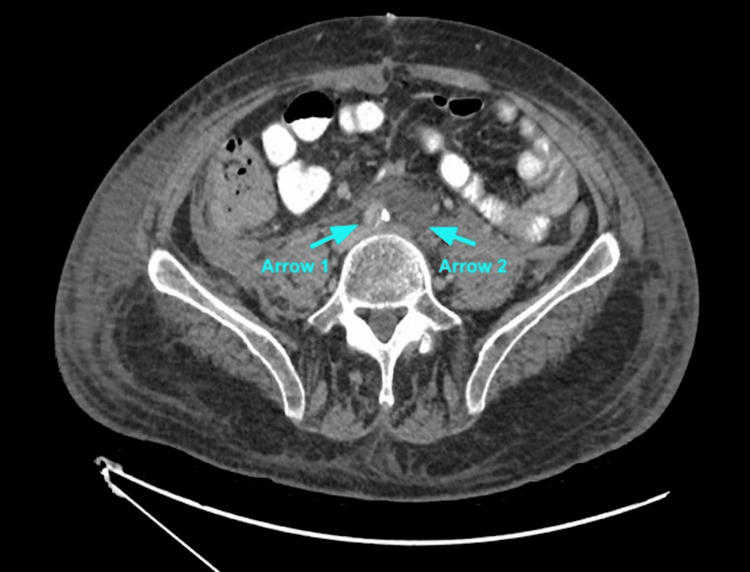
Severe Stenosis of the Right Common Iliac Artery and Occlusion of the Left Common Iliac Artery on CT Urogram Arrow 1: right CIA; Arrow 2: left CIA
